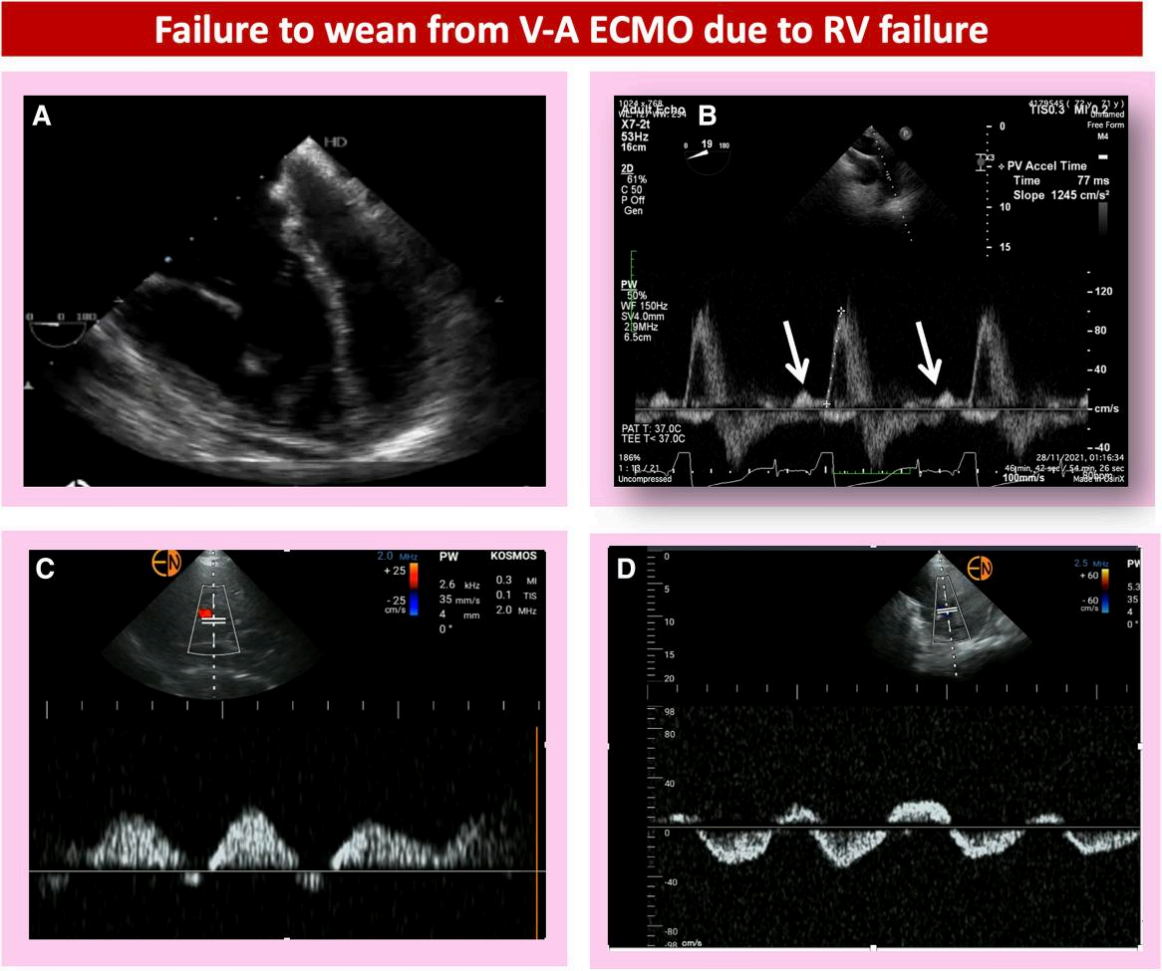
Echocardiographic and point of care ultrasound assessment of the right heart in a patient who failed weaning from V-A ECMO. (*A*) Mid-oesophageal four-chamber view at 0° showing significantly dilated RV with shift of the interatrial septum and interventricular septum to the left side. (*B*) Upper oesophageal ascending aorta view with pulsed wave Doppler interrogation of the PA showing shortened acceleration time (77 ms) and a pre-systolic A wave (white arrows), which indicates restrictive RV physiology in the context of elevated pulmonary vascular resistance. The pre-systolic A wave occurs when RV end-diastolic pressure exceeds PA diastolic pressure; hence, atrial contraction will lead to pulmonary valve opening. (*C*) Pulsed wave Doppler assessment of the portal vein in the same patient is showing significant portal vein pulsatility (>100%), which is a severely abnormal finding and indicates a significantly elevated RV preload (systemic venous congestion). (*D*) Pulsed wave Doppler assessment of the hepatic vein in the same patient is showing systolic flow reversal, which is a severely abnormal finding and indicates a significantly elevated RV preload (systemic venous congestion).
